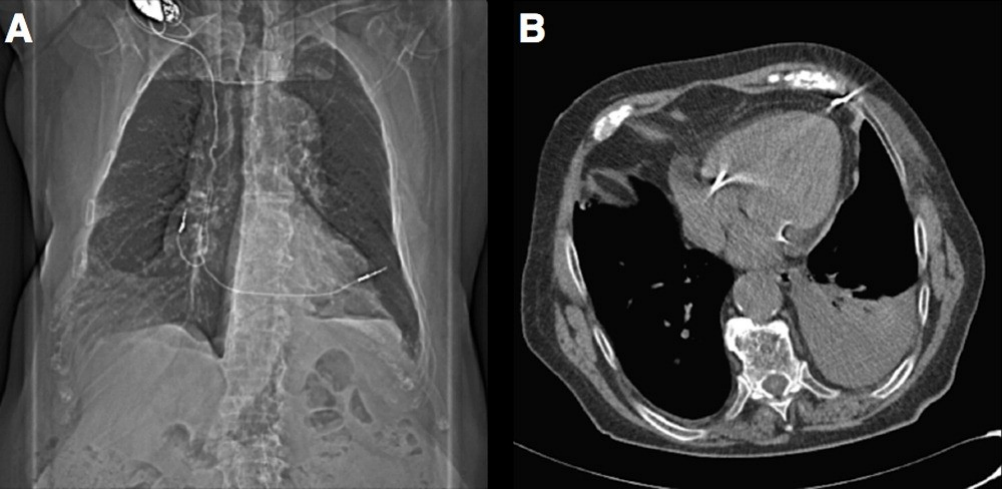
Cardiac perforation. (A) Chest computed tomography showing lead
